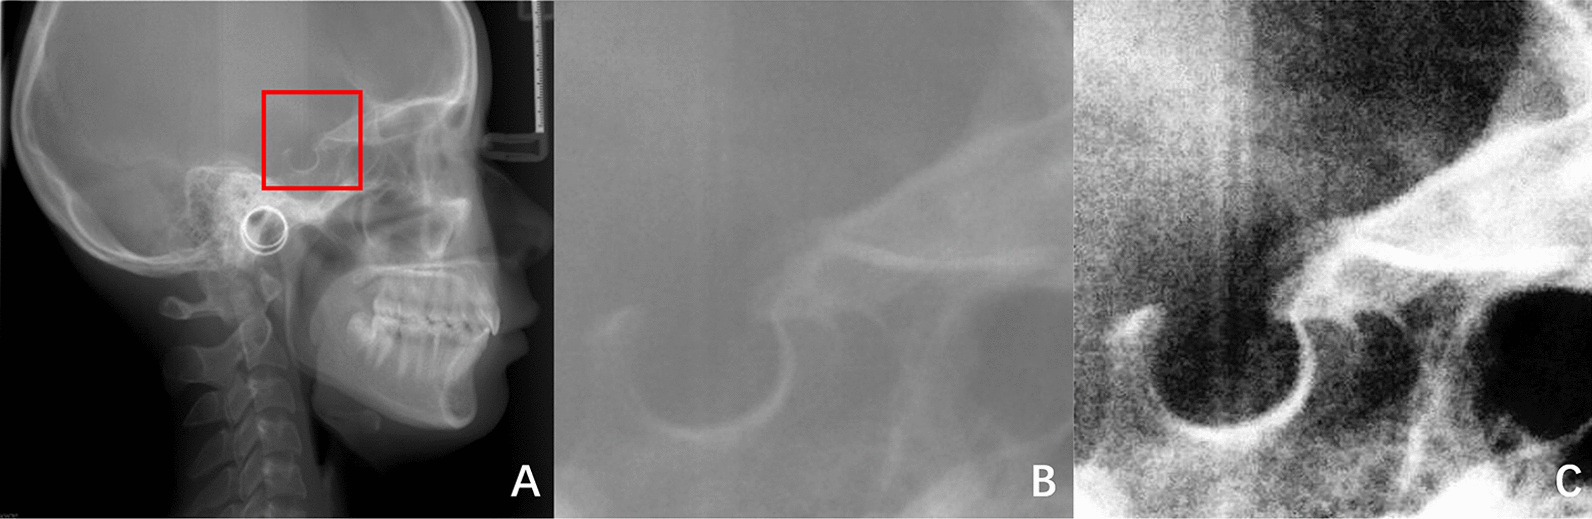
An example of image preprocessing. A An original X-ray image. B Result of extracting ROI. C Result of histogram equalization and median filtering
